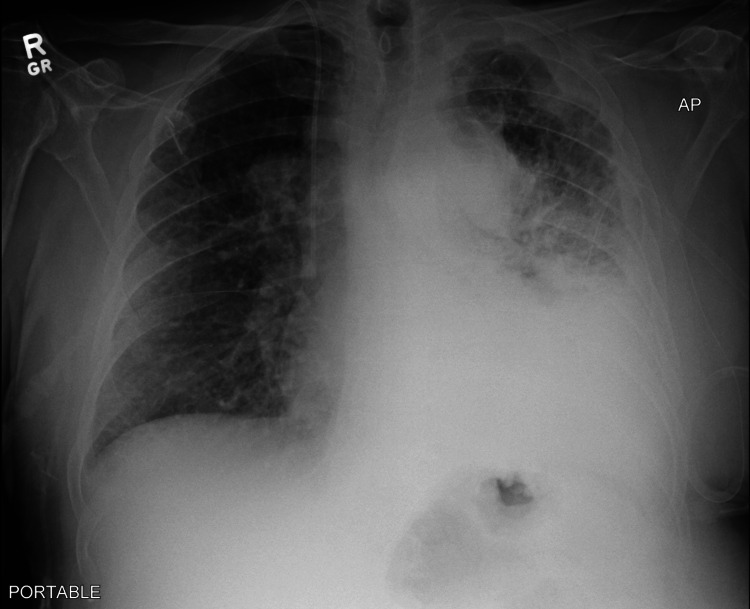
Initial chest radiograph showing large, left-sided opacity consistent with a moderate, left-sided pleural effusion and an underlying infiltrate with atelectasis There were also increased densities in the left upper lobe as well. In addition, extensive mediastinal lymphadenopathy was noted, as demonstrated by the right paratracheal widening.
